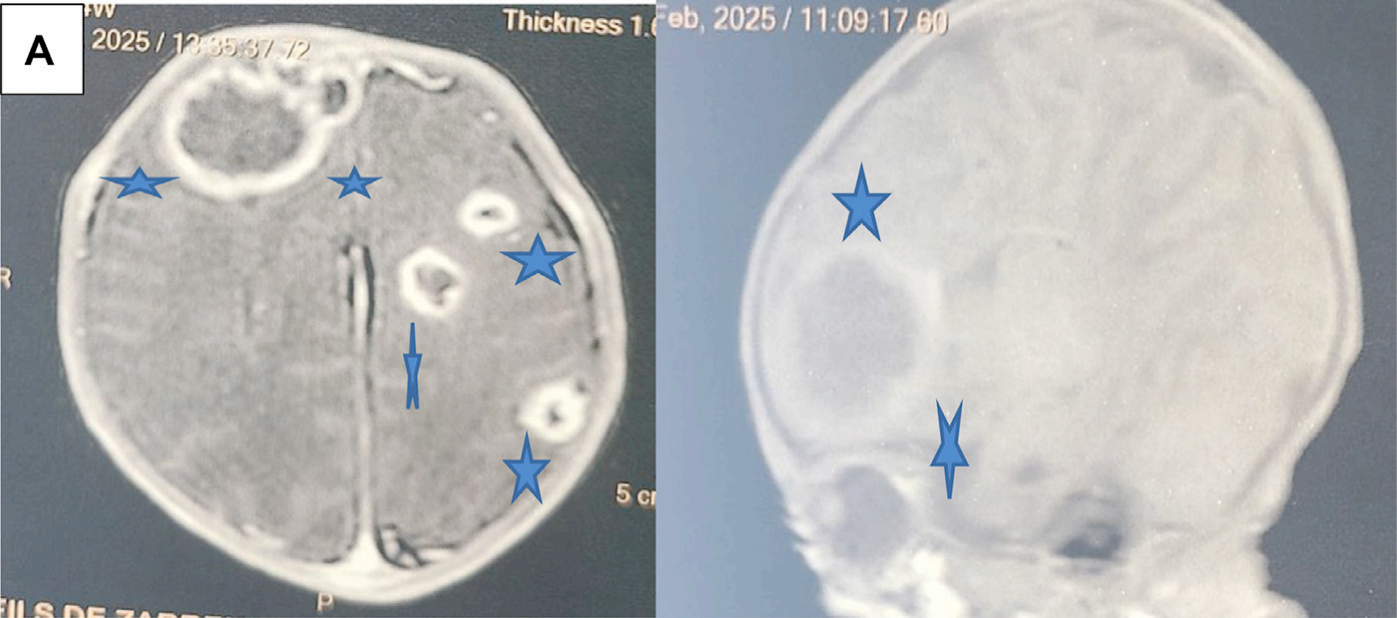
(A) Magnetic resonance imaging showed large cystic lesion in right frontal, parietal, temporal and occipital lobes. The largest lesion on the right side measured 2,3×1.3 cm and (B) Magnetic resonance imaging showed large cystic lesion in right frontal lobe.
